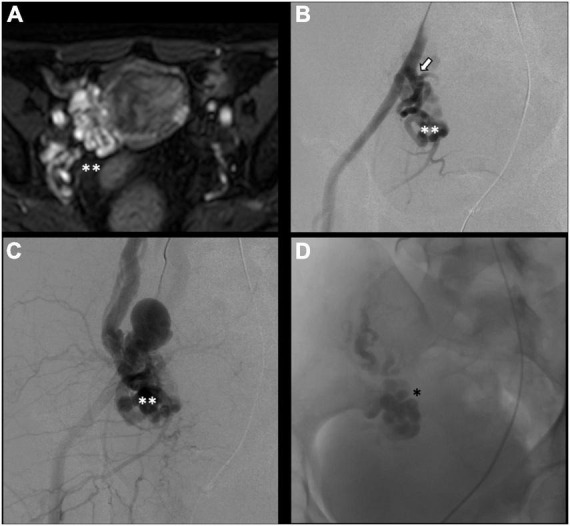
Type IV pelvic varices in a 44 yo female due to arteriovenous fistula. (A) Magnetic resonance imaging (MRI) shows right-sided pelvic varices (double white asterisks) with flow voids and early opacification in arterial phase. (B–D) Conventional phlebography shows early arterial opacification of varices (double white asterix) due to a fistula from the left internal iliac artery (white arrow), treated with Onyx embolization (single black asterisk).
